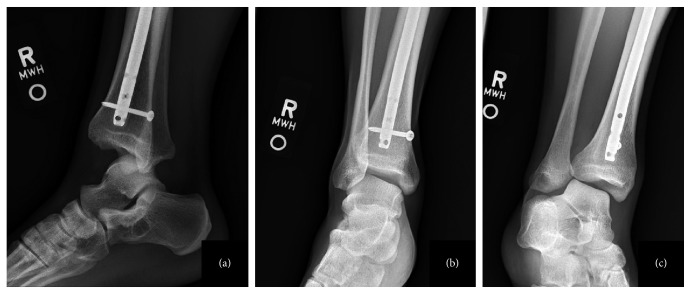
Preoperative lateral (a), AP (b), and mortise (c) radiographs showing tibiotalar dislocation and impingement of the fibula behind the tibia with no fractures.
